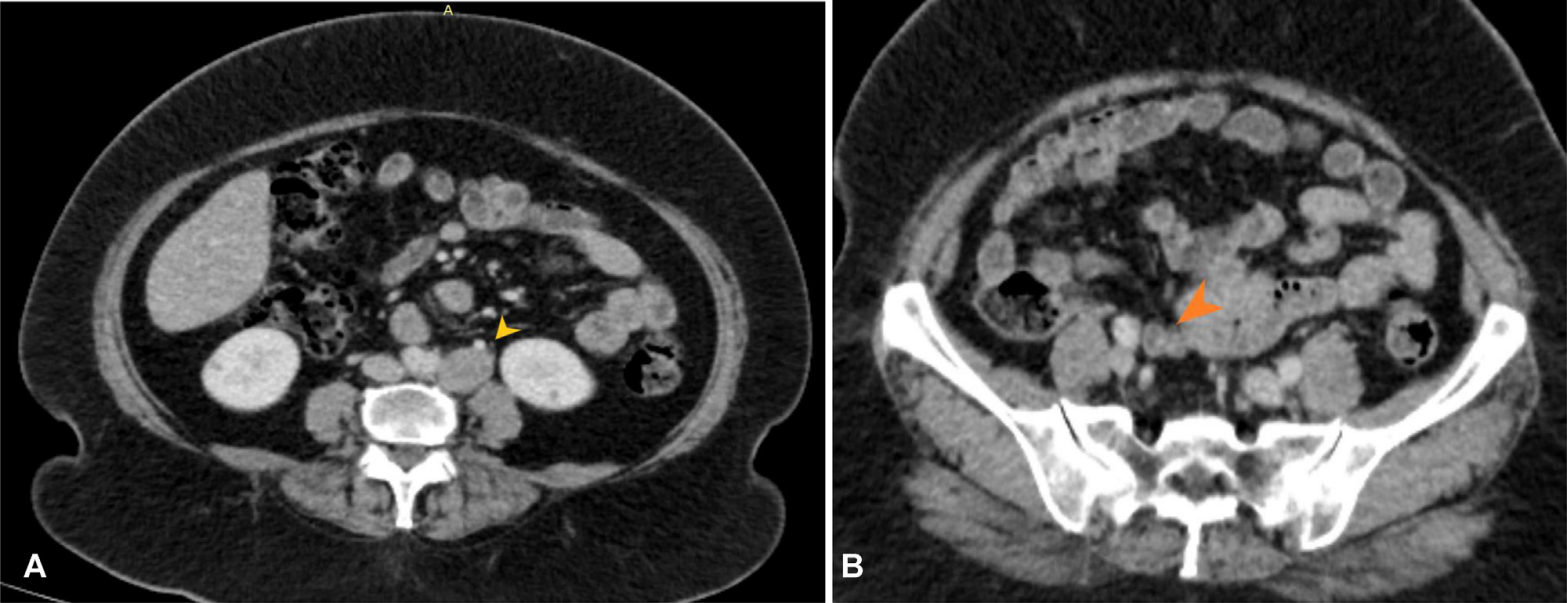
CT abdomen (soft tissue window) in axial plane show enlarged left para aortic (A, yellow arrowhead) and right common iliac lymph nodes (B, orange arrow head).
